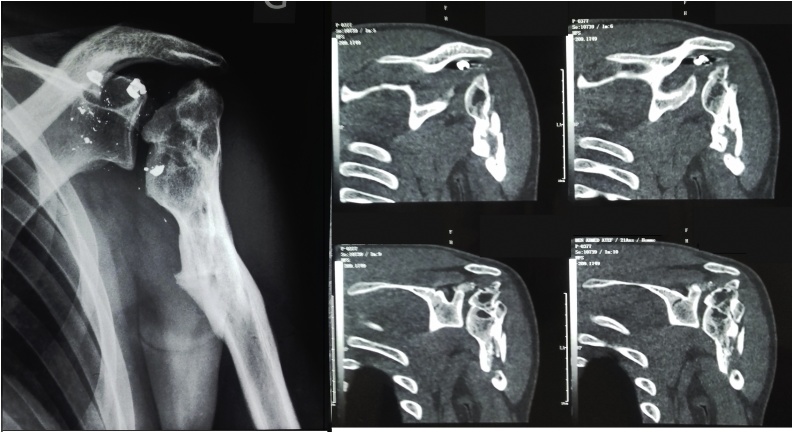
Standard radiography and CT scan showing bone malunion with a total loss of the hemispherical form of the humeral head.
